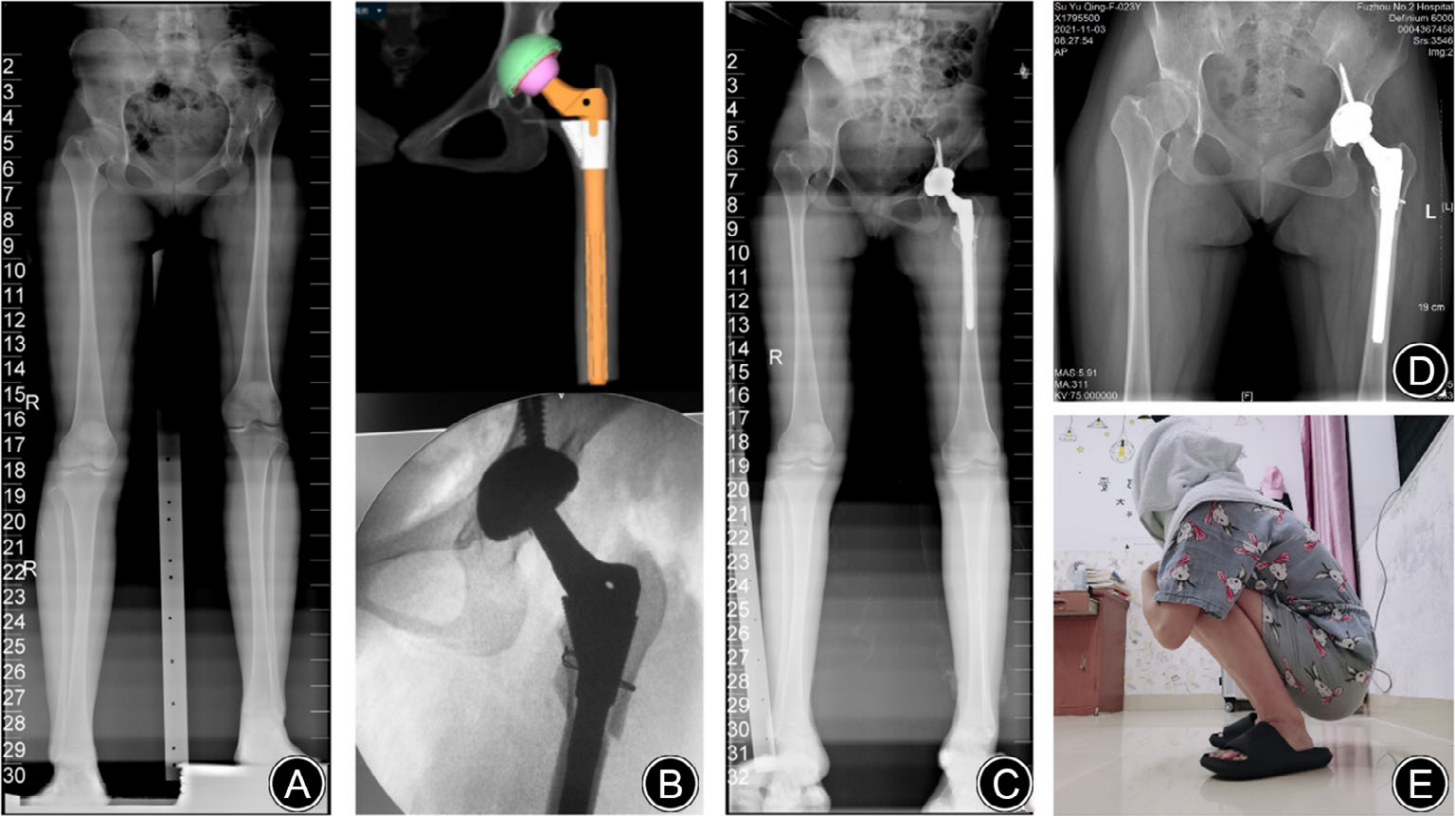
Case presentation: DAA‐THA for unilateral hip dysplasia in 22‐year‐old female: (A) preoperative full‐length X‐rays of the lower limb; (B) the comparison between preoperative AI planning and intraoperative X‐ray fluoroscopy; (C) postoperative full‐length X‐rays of the lower limb; (D) anteroposterior radiograph of pelvis 1 year after operation; (E) the hip activity at last follow‐up.
